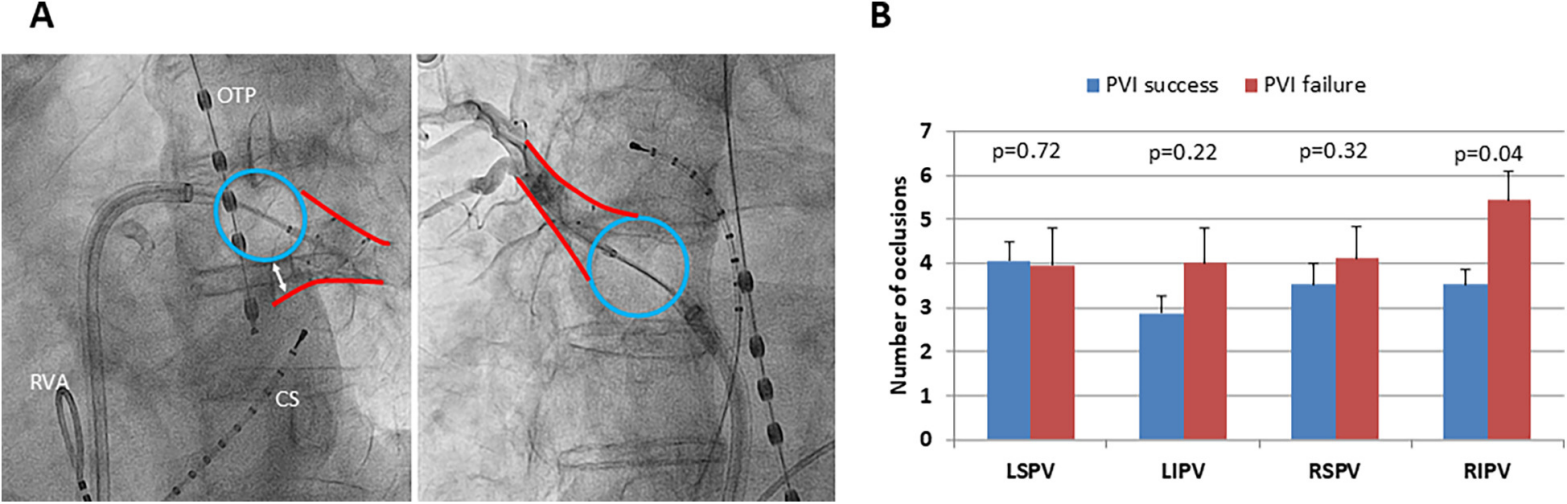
Number of occlusions and freedom from AF recurrence following PVI. (A) Left side: x-ray (LAO 50°) of occlusion grade 1 with significant reflow of contrast agent due to poor balloon occlusion of the left inferior pulmonary vein. Right side: x-ray (RAO 30°) of occlusion grade 3 with no reflow of contrast agent due to optimal balloon occlusion of the right superior pulmonary vein. Blue circle: inflated cryoballoon, the red outlines mark the outer borders of the particular pulmonary vein, and the white double arrow marks the extent of poor occlusion. OTP, esophageal temperature probe; CS, coronary sinus catheter; RVA, right ventricular apex catheter. (B) Mean amount of occlusion attempts for each pulmonary vein to reach optimal occlusion grading, distinguished between successful pulmonary vein isolation (PVI success, blue bars) and AF recurrence (PVI failure, red bars) 1 year following index procedure; the black lines mark standard error of the mean. LSPV, left superior pulmonary vein; LIPV, left inferior pulmonary vein; RSPV, right superior pulmonary vein; RIPV, right inferior pulmonary vein. p* < 0.05.
